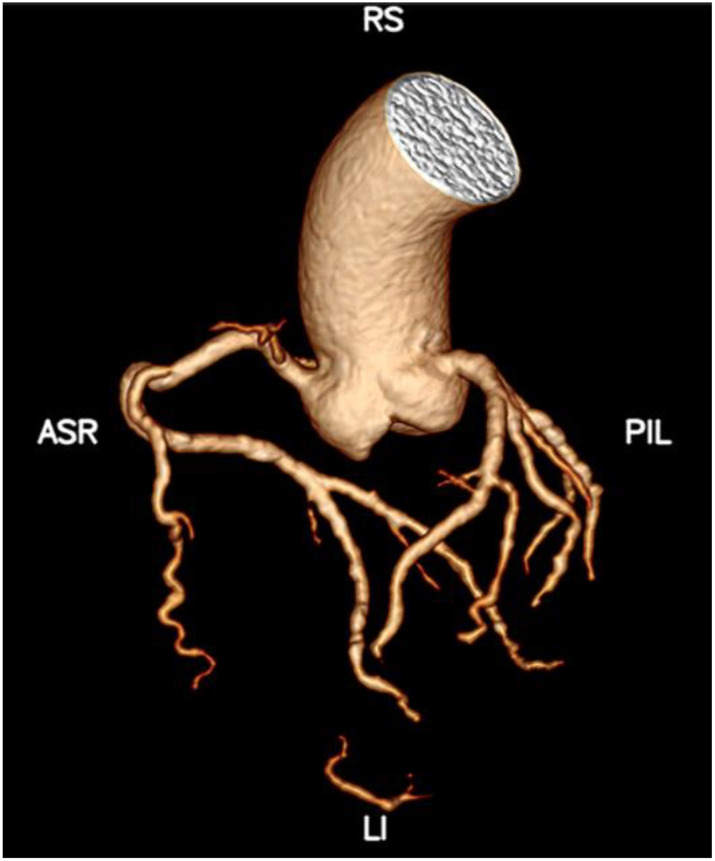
3D reconstructed coronary CTA images.
